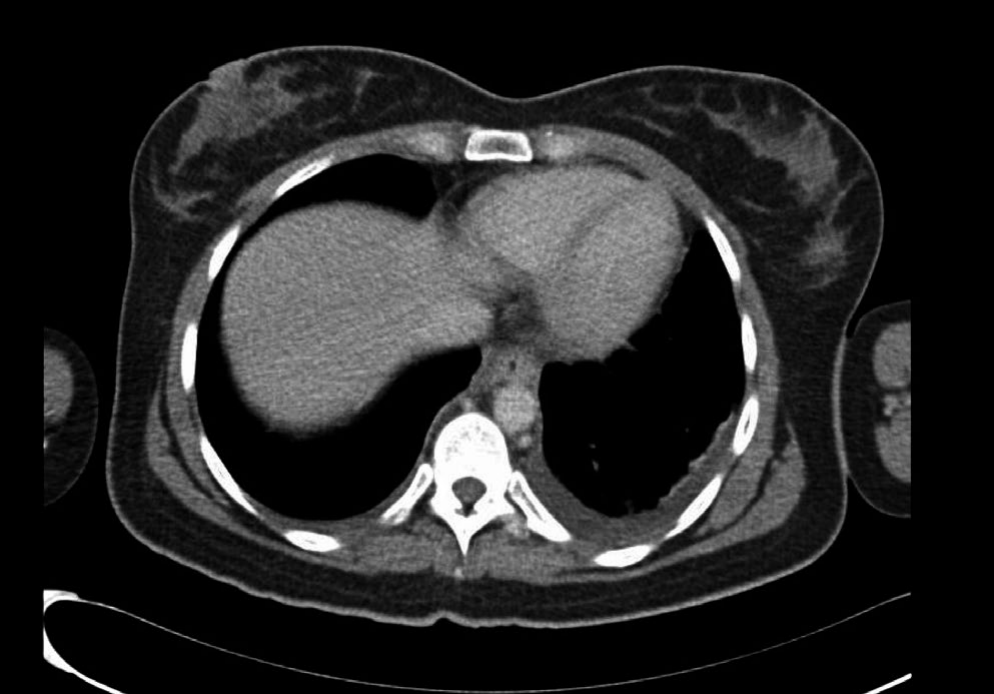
CT scan of the chest showing left‐sided pleural effusion with high attenuation suggestive of chylothorax
